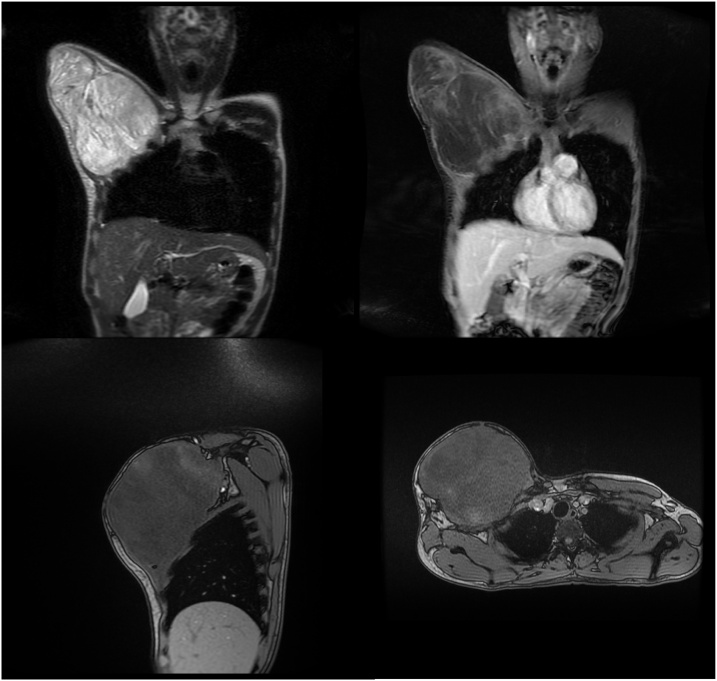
MRI results showing recurrent tumor.
